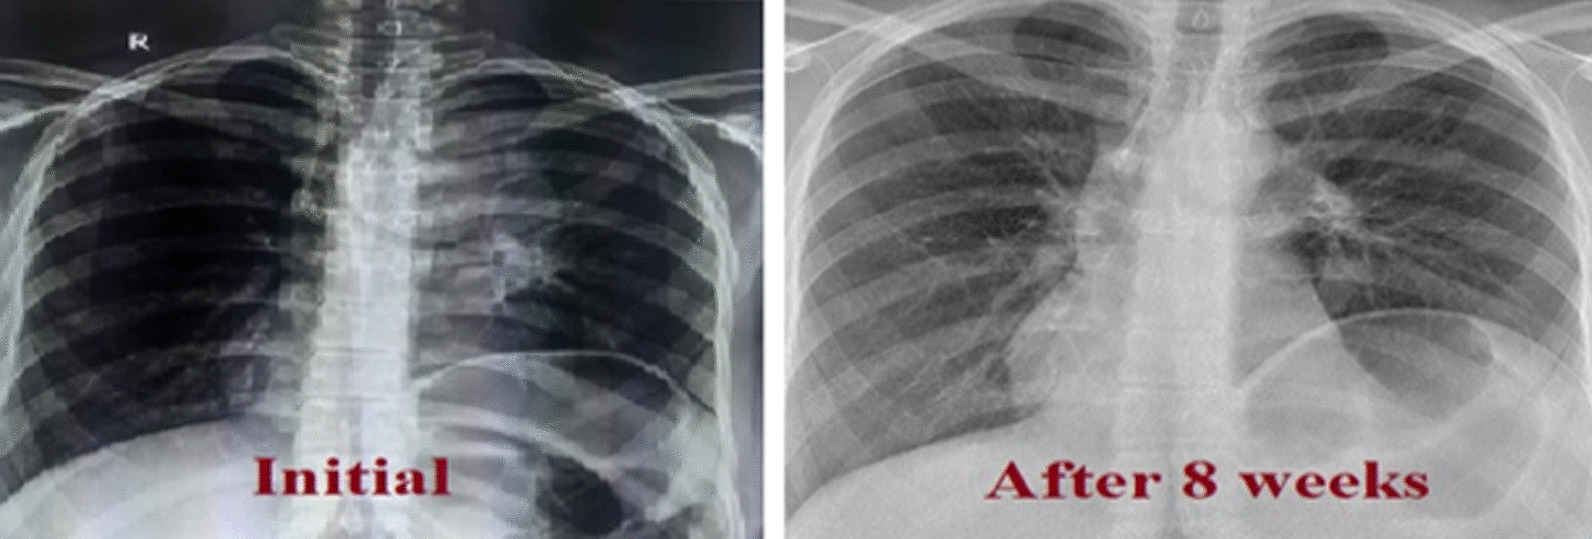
Chest X-rays showing prominent left bronchoalveolar marking and elevation of left hemidiaphragm
